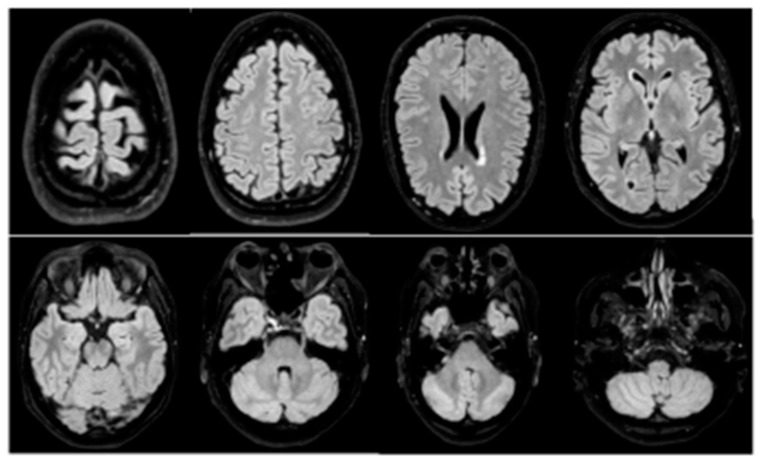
Gadolinium-based brain MRI: negative for brain metastasis or meningeal carcinomatosis. In particular, no appearance of signal alterations in the cerebral parenchyma both in the supratentorial and subtentorial sites. No areas of diffusion restriction to refer to recent ischemic lesions. After administration of gadolinium, no pathological enhancement of the cerebral parenchyma or meninges. Unchanged morphology and dimensions of the ventricular system. Structures of the median line in axis.
